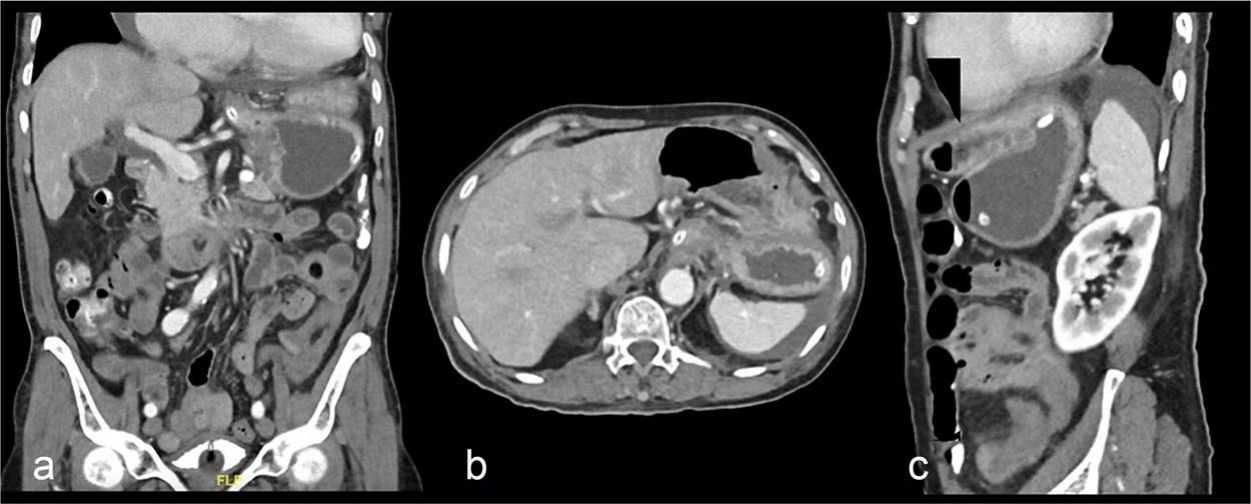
Contrast-enhanced CT abdomen and pelvis shows nasogastric (NG) tube noted in position. The stomach demonstrates abnormal orientation with inferior displacement of the fundus and superior displacement of the gastric pylorus near the gastroesophageal junction, with interval improvement of the gastric distension, likely mesenteroaxial gastric volvulus. Persistent mild perigastric and perisplenic fluid. Preserved gastric wall enhancement. Mild pelvic free fluid is noted. No free air or size significant lymph node.
